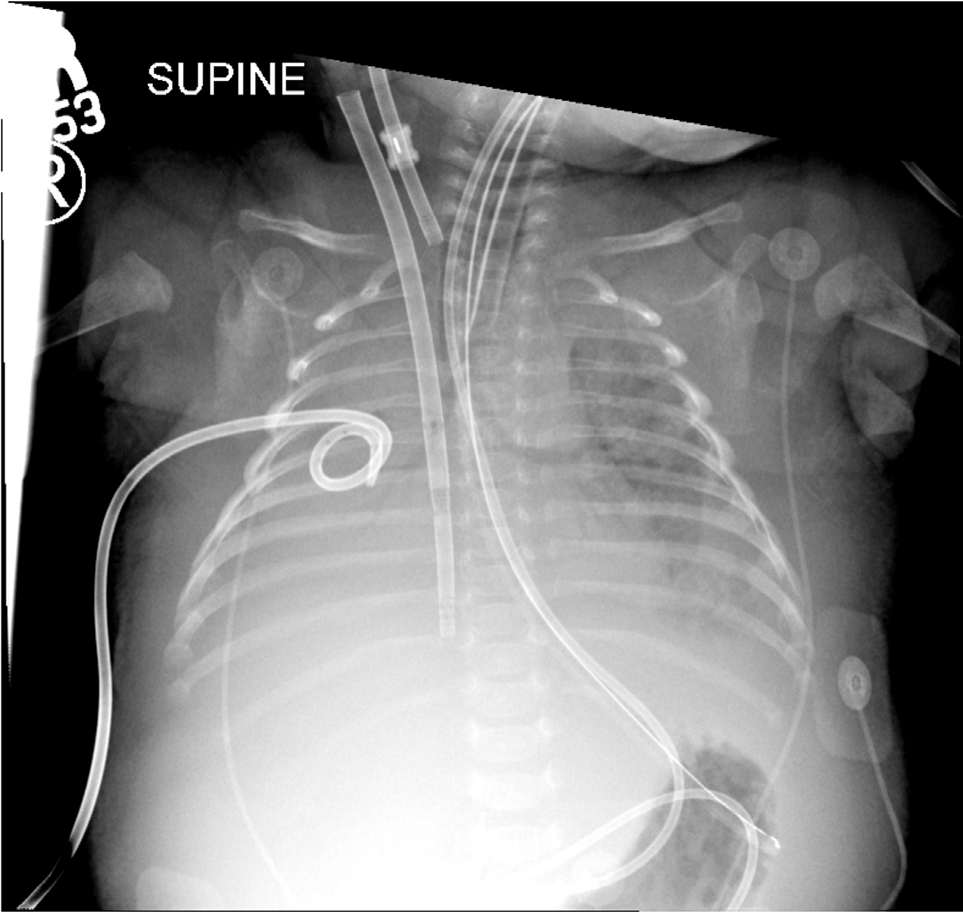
Chest radiographs before initiation of intrapulmonary percussive ventilator therapy and 4 hours after bronchoscopy. There was improvement of aeration of the left lung with persistent hazy opacifications in the right upper and middle lobes.
